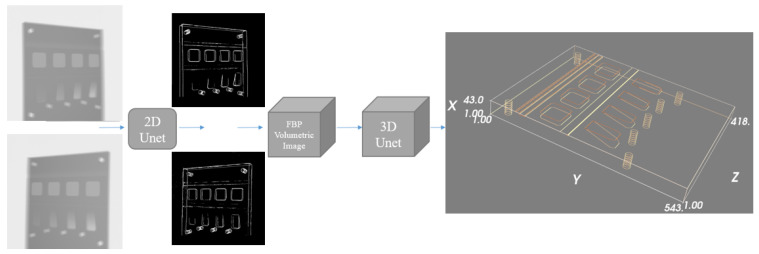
Overview of the proposed framework. The input is a pair of X-ray projection images. Each projection is fed independently into the same 2D U-net to compute two different feature maps, where the background is removed, leaving estimates of the line and point feature locations. Utilising scan geometry knowledge, the two feature maps are then back-projected into a 3D volume using the FDK algorithm, with the back-projected volume further processed using a 3D U-net to generate the 3D spatial feature maps.
